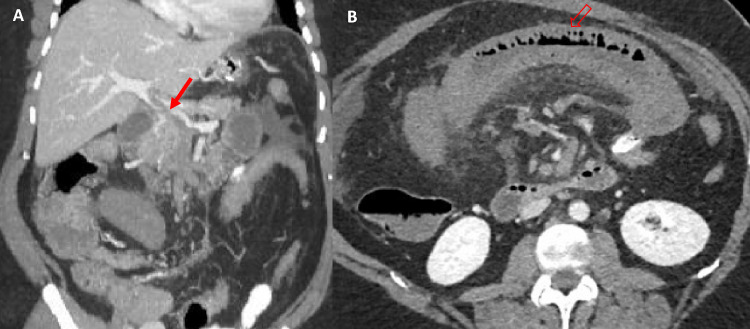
Case 1. A Coronal MIP image of a CT examination showing partially occlusive portal and splenic vein thrombus and occlusive superior mesenteric vein thrombus (solid arrow). B Axial image from the same CT showing an oedematous and dilated loop of jejunum in keeping with ischaemia (unfilled arrow)
